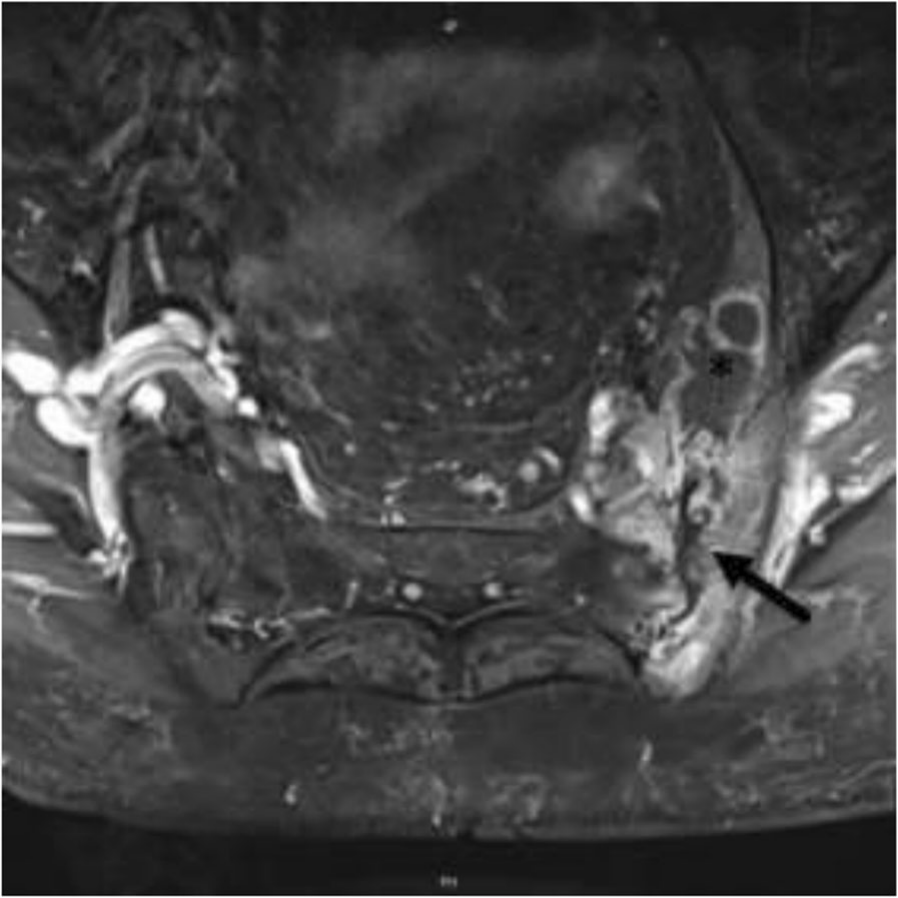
Abdominal magnetic resonance image showing destruction of the left iliac joint with surrounding edema (arrow) and a small collection of fluid ventral to the iliosacral joint (star)
